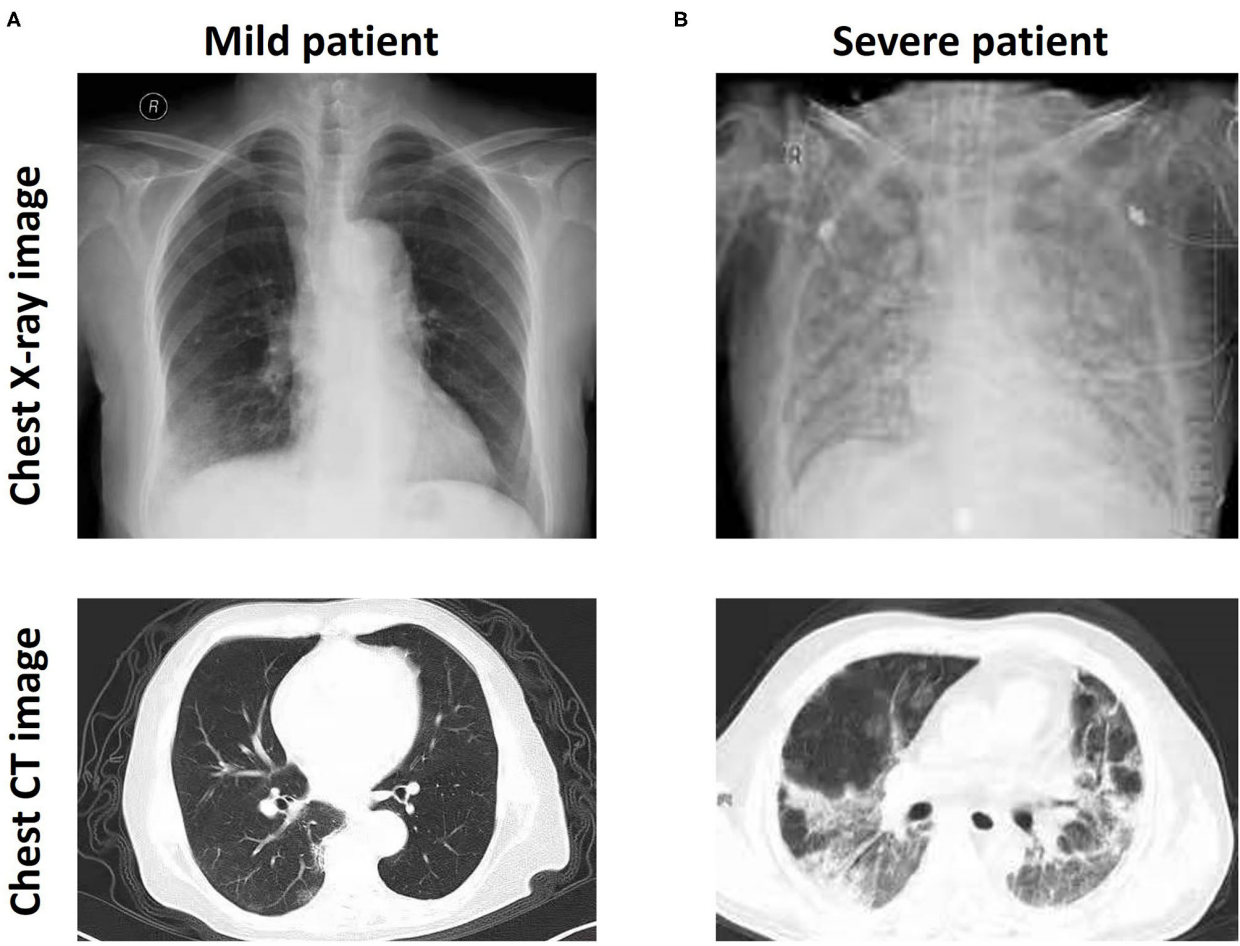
CT and bedside chest X-ray images of patients with mild and severe COVID-19. Bedside chest X-ray and chest CT images showing multiple patchy shadows and ground glass opacity in the lungs of patients with mild (A) and severe (B) COVID-19.
